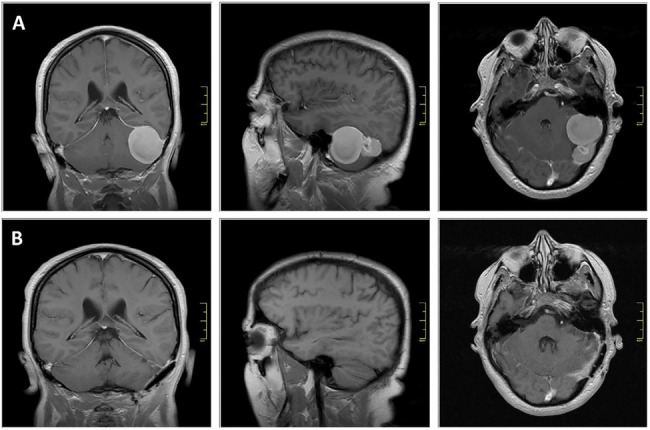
Meningioma in a patient with Cowden syndrome A. Coronal (left), sagittal (middle) and axial (right) gadolinium- enhanced T1-weighted MR images at pre-operative stage. B. contrast enhanced T1-weighted MRi images following neurosurgical intervention. Scale bar represents 4 cm.
